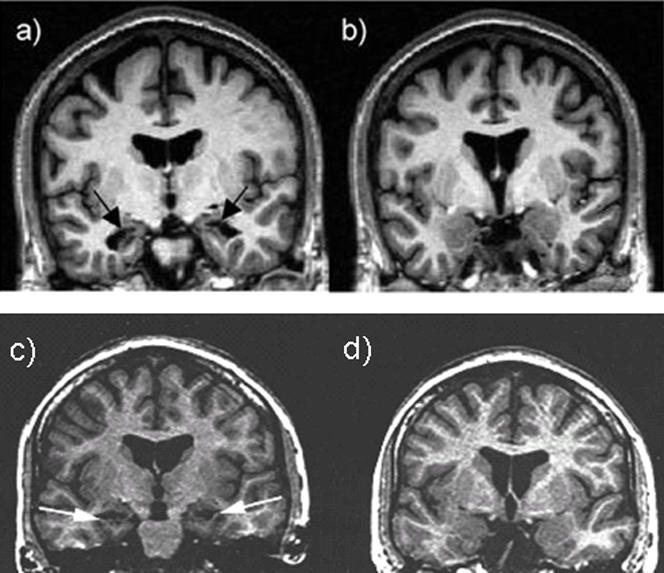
Anatomical images of amnesic patients. -T1 weighted coronal MRI sections of two of the patients35,36. Images reveal selective bilateral atrophy of hippocampus (a & d), and intact amygdala (b & c).
